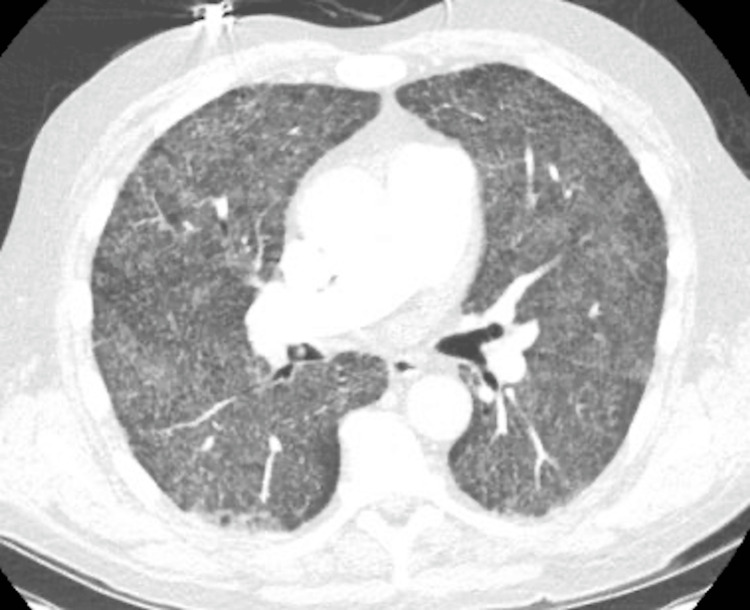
CT chest of case 4 A representative cut from the CT Chest showing diffuse ground-glass opacities and interstitial thickening
